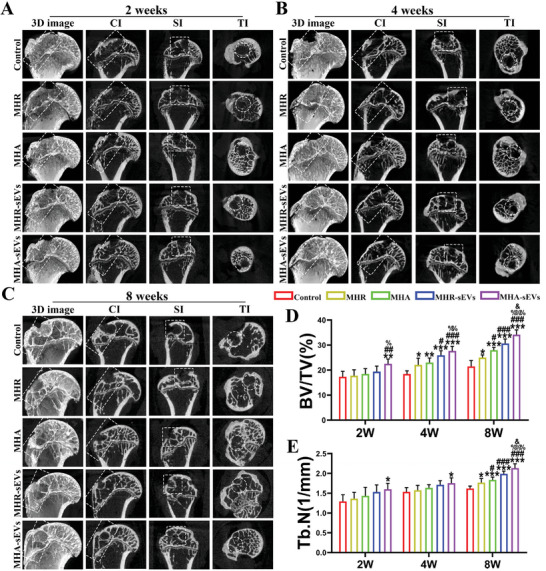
MHA‐sEVs enhance new bone formation in the bone tunnel of the humeral head. A–C) Representative 3D reconstruction, coronal, sagittal, and transverse μ‐CT images at 2, 4, and 8 weeks of tendon repair. D,E) Semi‐quantitative analysis of BV/TV and Tb.N of regenerated bone tissue. The bone tunnel area was circled by white dotted lines. CI, coronal images; SI, sagittal images; TI, transverse images. ^*^
